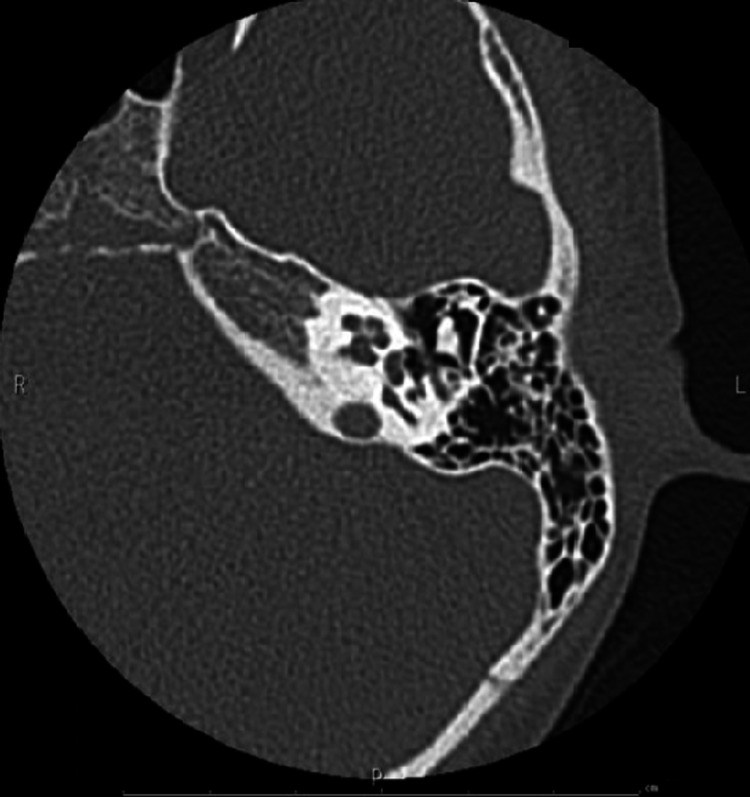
Imaging of Case 2: temporal bone CT (left) Affected side. CT, computed tomography.
